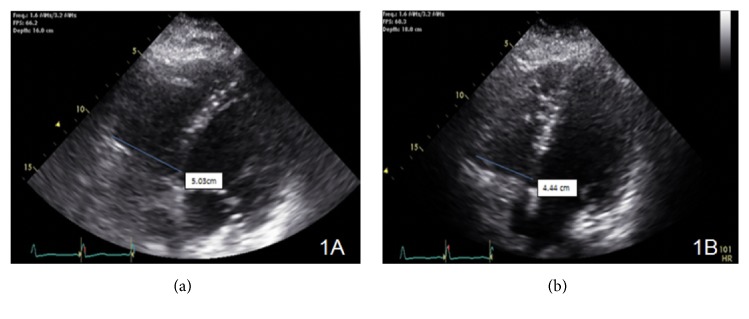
(a) Right ventricular size and function assessment was done with RV focused imaging from apical four chamber view. Initial measurement showed 5.03 cm RV basal diameter consistent with moderately dilated RV size. 2D images also showed reduced RV ejection fraction along with RV strain findings. (b) Right ventricular size and function assessment was done with RV focused imaging from apical four chamber view. RV basal diameter is measured as 4.44 cm which was consistent with mildly dilated RV size. RV ejection fraction is improved, and RV strain findings were no longer available.
